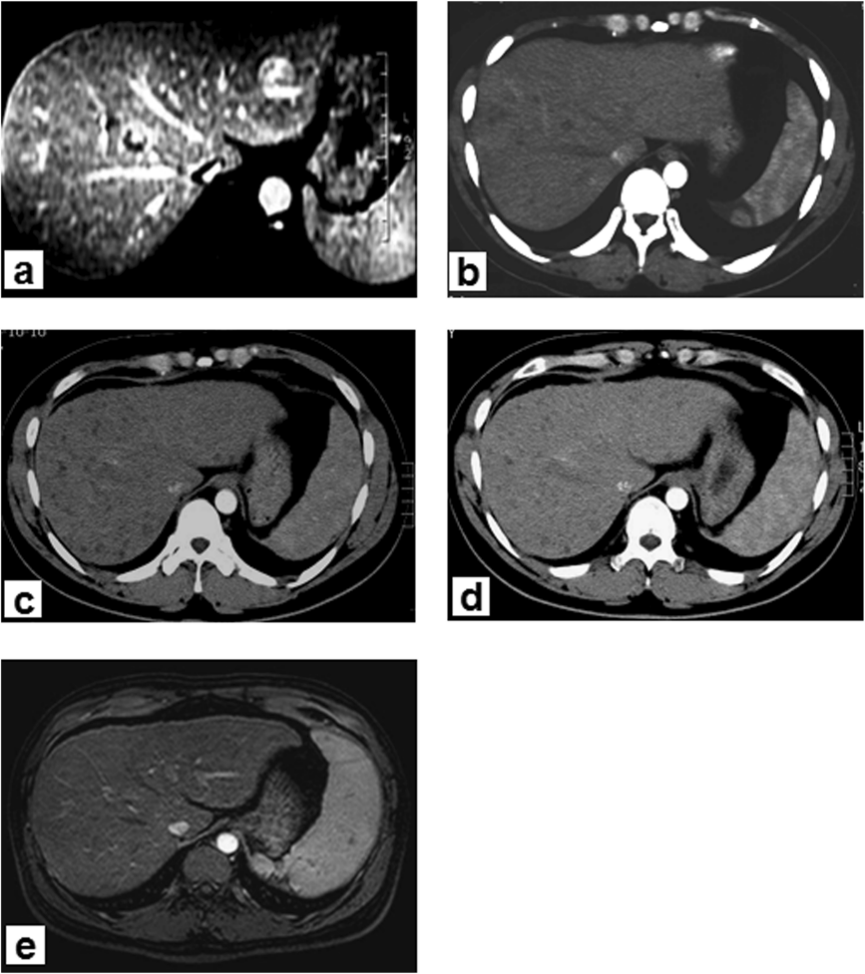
Abdominal computed tomography (CT) or magnetic resonance imaging (MRI). (a) Abdominal dynamic enhanced MRI obtained before treatment shows multiple liver lesions, the number of nodules >100, the largest diameter was about 1.55 cm; AFP level, 4,773 ng/ml. (b) CT scan obtained 2 months after the start of treatment showed that the lesion was not enhanced in the arterial phase; AFP level, 3.79 ng/ml. (c) CT scan obtained 15 months after the start of treatment showed that the number of low-density lesions was less than before, also no enhancement; AFP level, 3.63 ng/ml. (d) CT scan obtained 21 months after the start of treatment showed that the diffuse liver lesions disappeared, no abnormal enhancement lesions; AFP level, 2.26 ng/ml. (e) MRI scan obtained 46 months after the second treatment showed that there were still no lesions in the liver; AFP, level 3.73 ng/ml.
